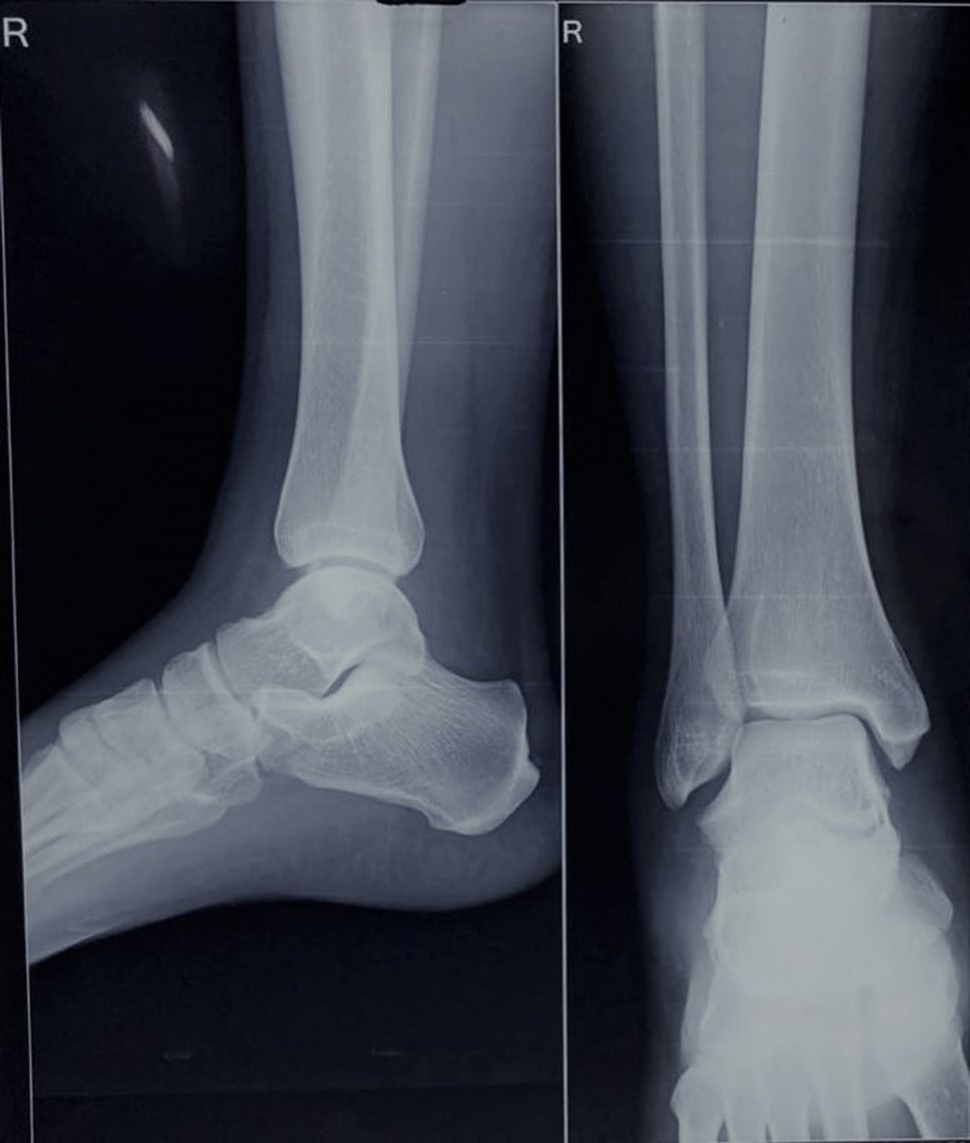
Antero-posterior and lateral Radiograph of the ankle joint. Osseous anatomy is normal
